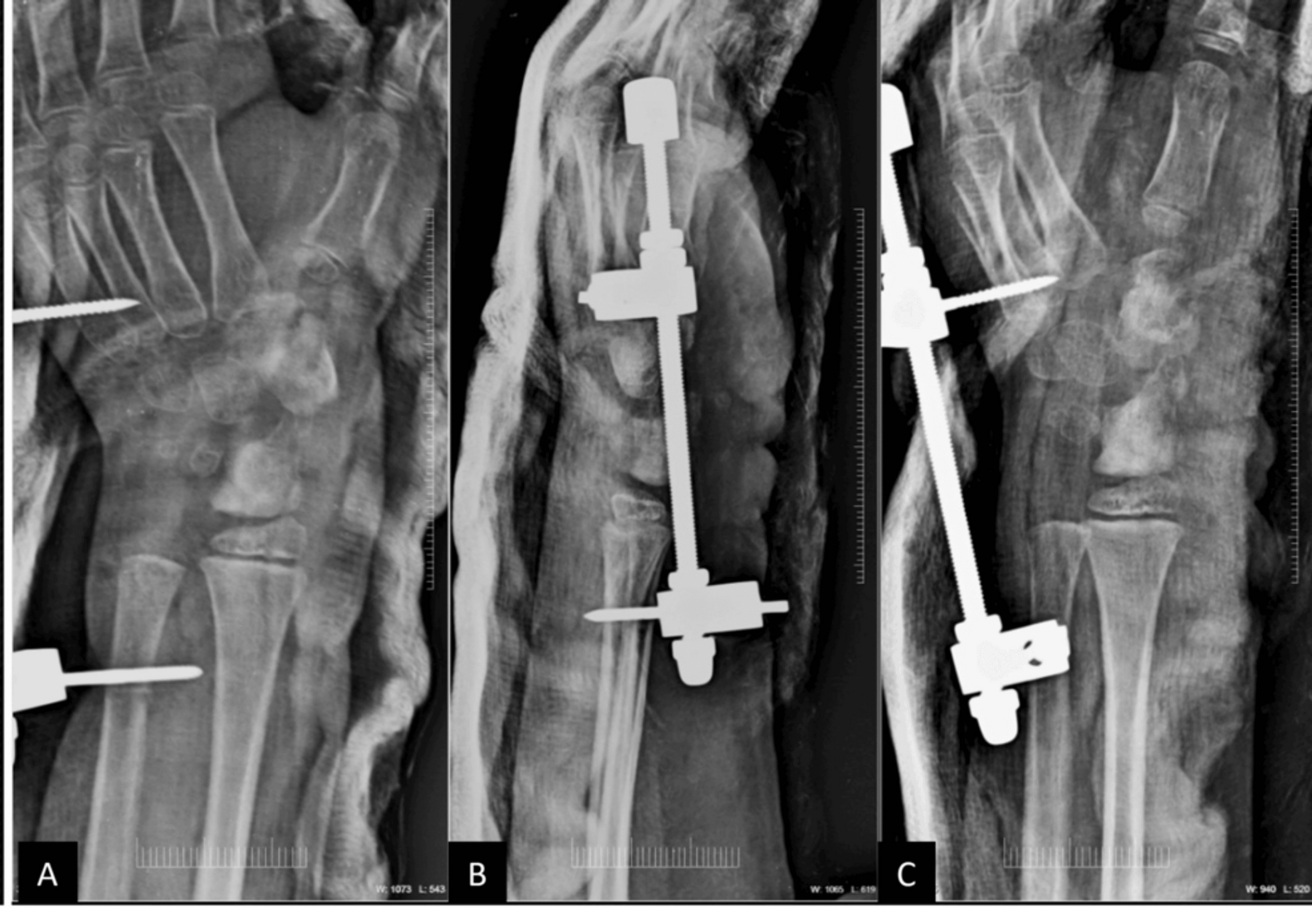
Postoperative X-rays. (A) AP view, (B) lateral view, and (C) oblique view showing reduced size of the carpal bones following tumor debulking, with removal of the lesion in the abductor pollicis longus tendon sheath and placement of the distraction apparatus.
